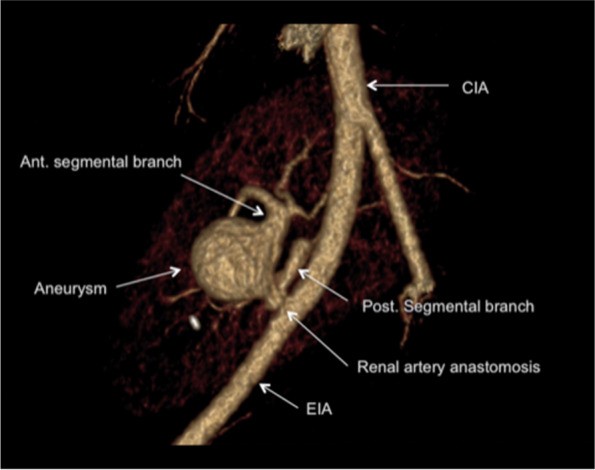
3D reconstruction image of the CT Angiogram showing TRAA arising from the anterior segmental branch
